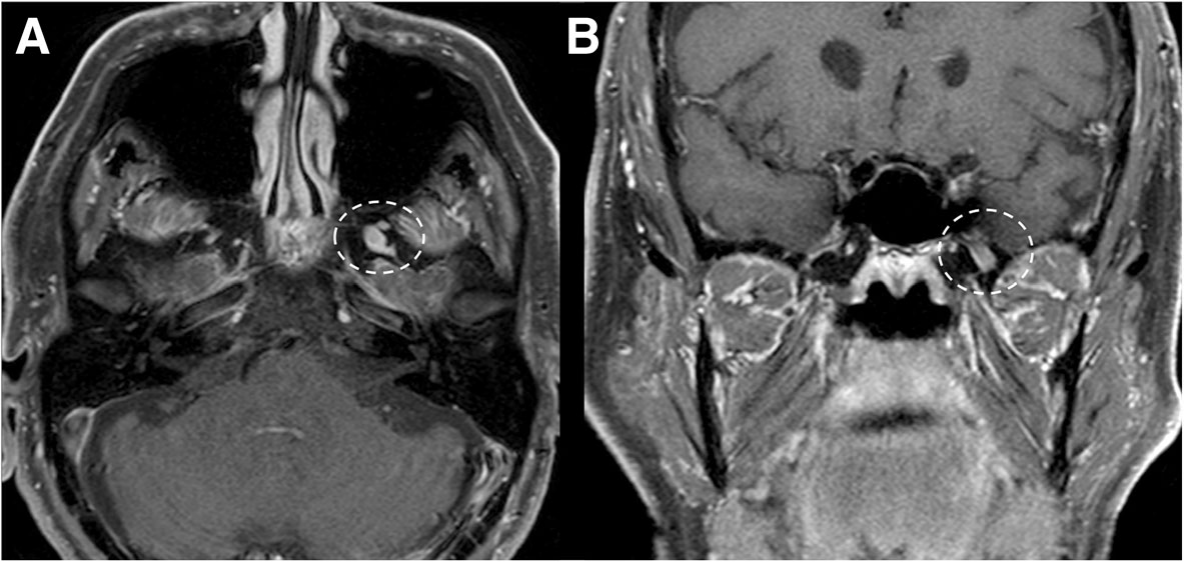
Perineural spread of tumor. MRI T1-weighted post-gadolinium axial (a) and coronal (b) images. Note the enlargement and the enhancement of the mandibular branch of trigeminal nerve (V2) (dotted circles)
